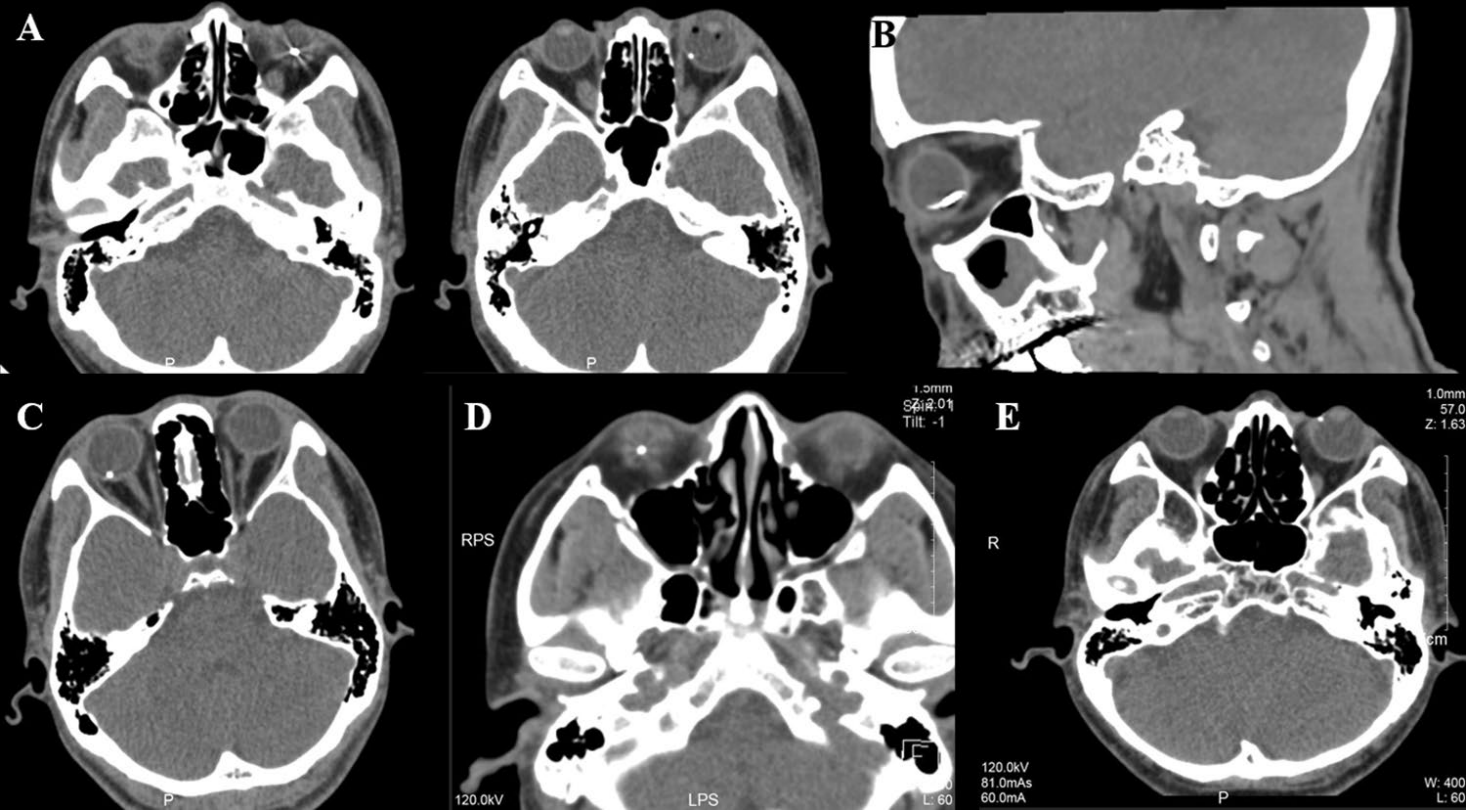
(A) Two foreign bodies in one eye on different layers of CT; (B) large foreign body of 15 mm × 3 mm; (C) foreign body lay on retina; (D) foreign body in vitreous body; (E) foreign body near ciliary body.
